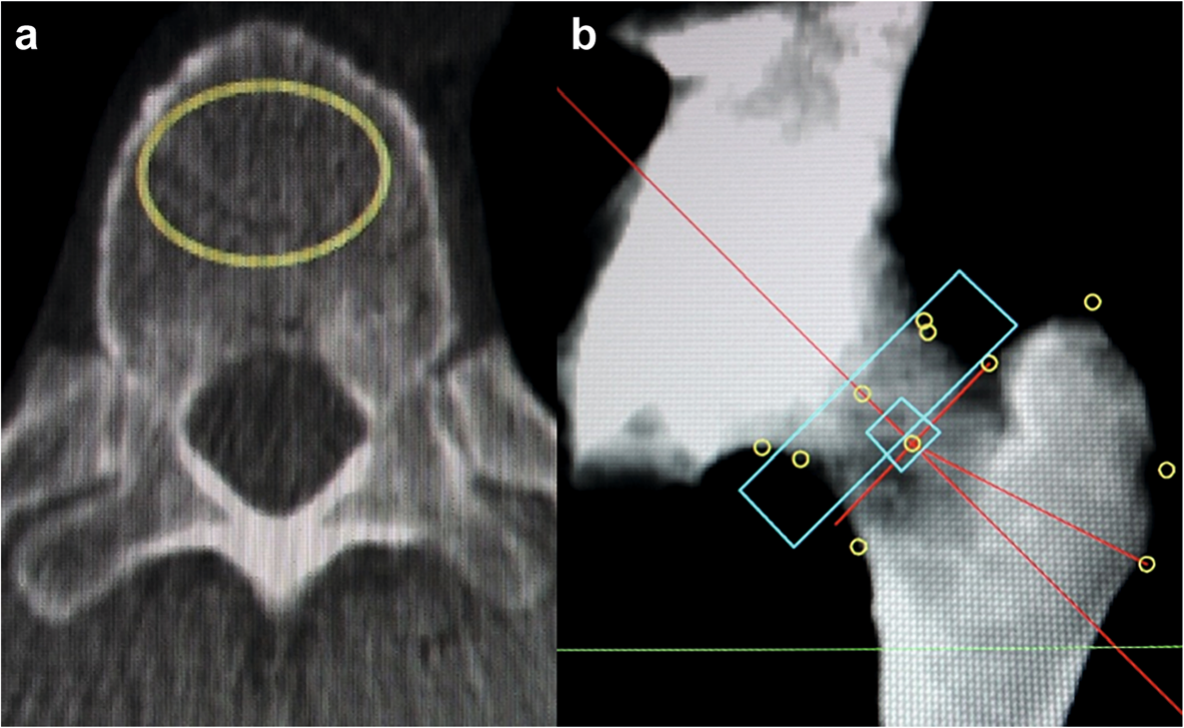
QCT BMD measurements. Examples for quantitative computed tomography bone marrow density measurements in the spine (a) and the femur (b)
